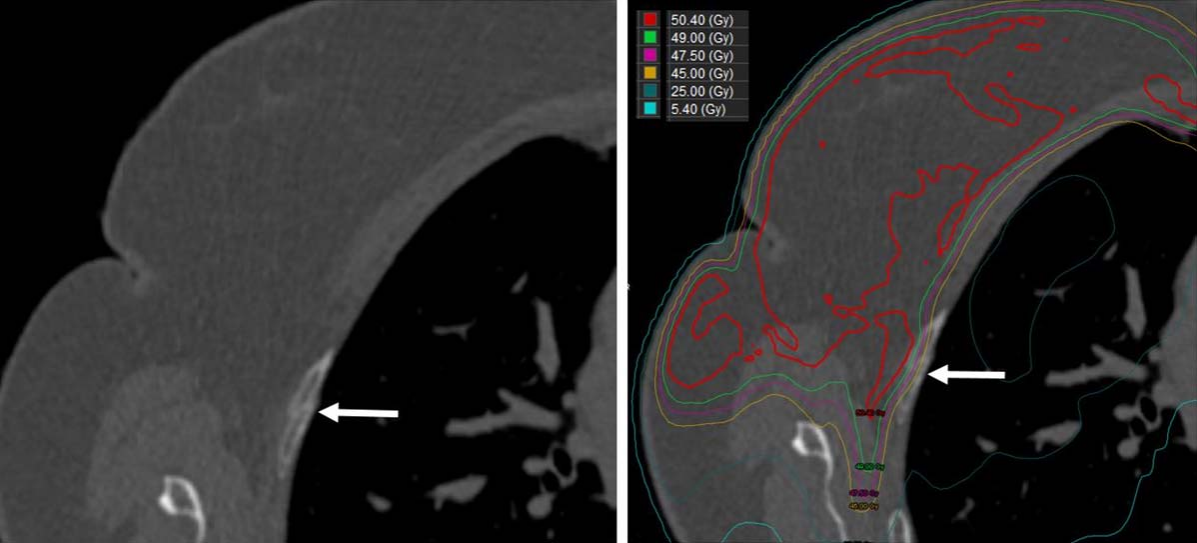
The right third rib was fractured (arrow) after the patient experienced a motor vehicle accident (left). The radiation dose was deformed to the CT that demonstrated this fracture (right). The D0.5cm^3^ dose to the fractured rib was 38.3 Gy. Abbreviations: CT, computed tomography; D0.5cm^3^, the minimal dose received by the highest irradiated volumes of 0.5 cm^3^.
